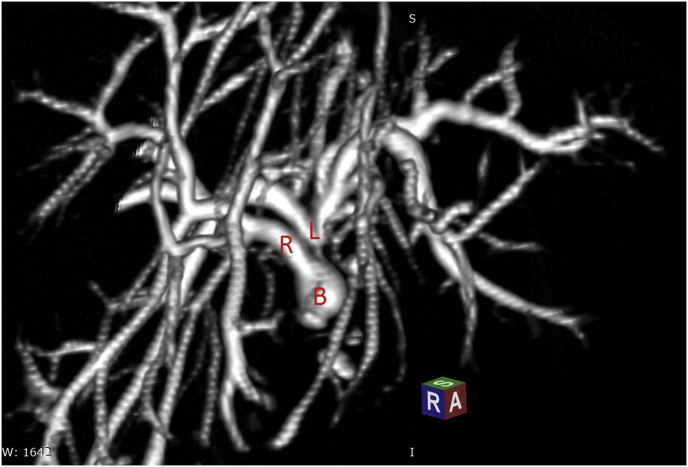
MRI with 3D reconstruction of a biliary stricture, B: Biloma , L = Left hepatic bile duct, R: Right hepatic bile duct.
